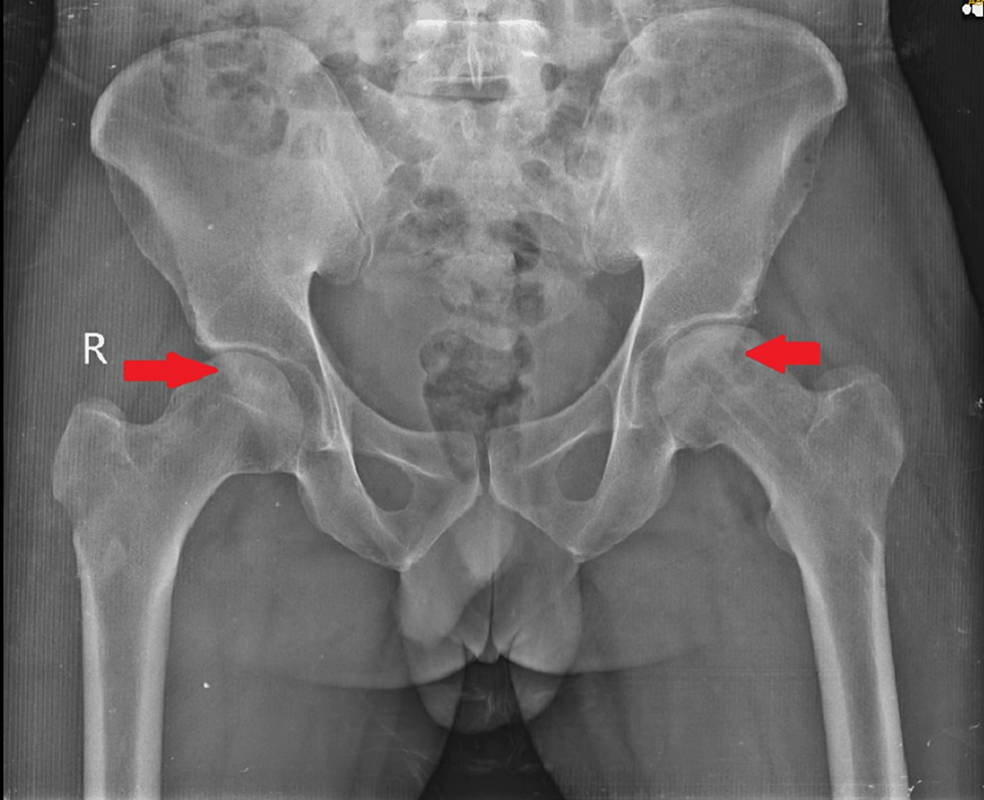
Post-operative AP radiograph of the pelvis showing core decompression with reverse bone grafting
